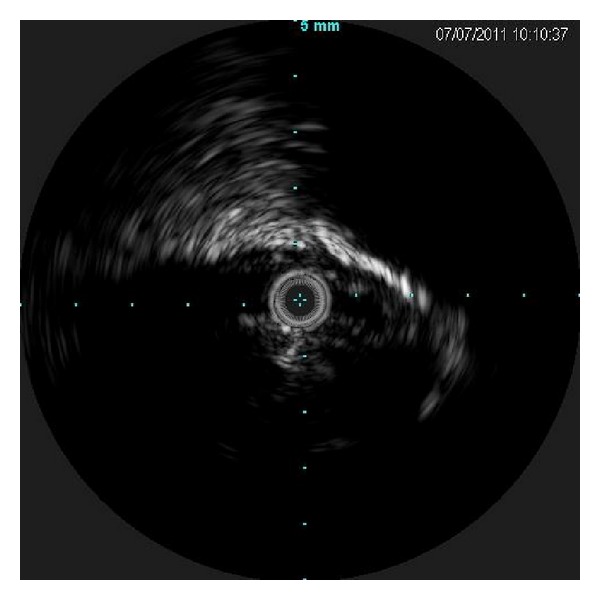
Intravascular ultrasound (IVUS) in the left iliac vein (anterior at top of image) demonstrating compression from the adjacent right iliac artery (2 o'clock position) before stenting.
